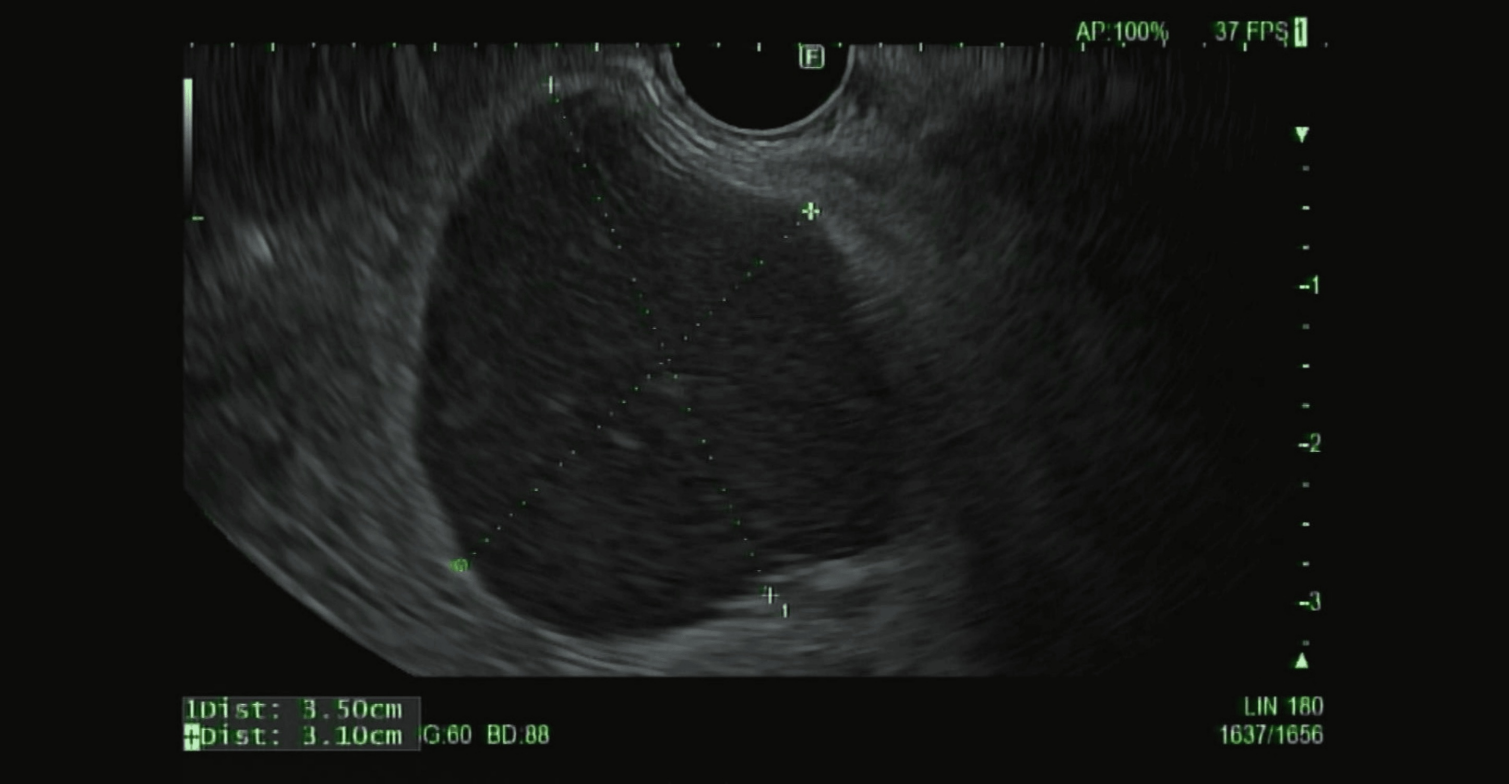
Endoscopic ultrasound image of the mass with dimensions
